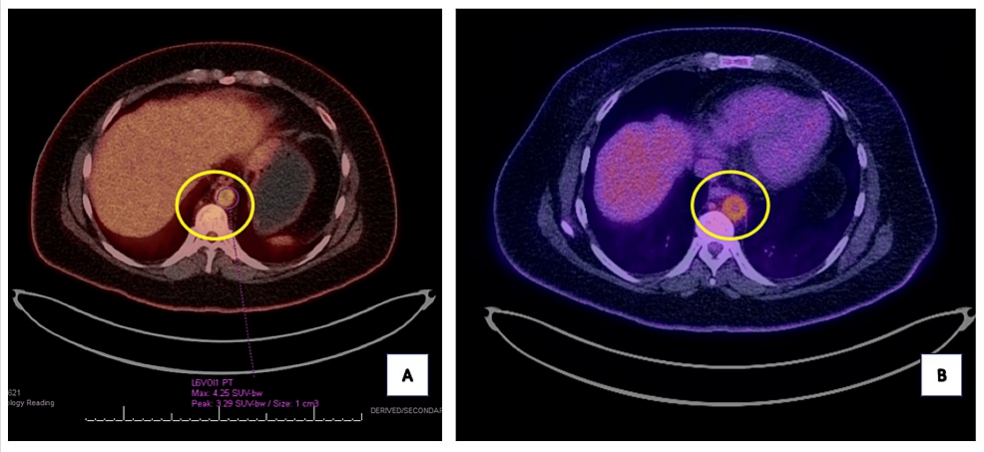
Positron emission tomography CT scan of the abdomen and pelvis. The yellow circles in A and B show significant metabolic activity in the descending aorta to subclavian arteries, iliofemoral, and popliteal arteries, indicating active vasculitis.
